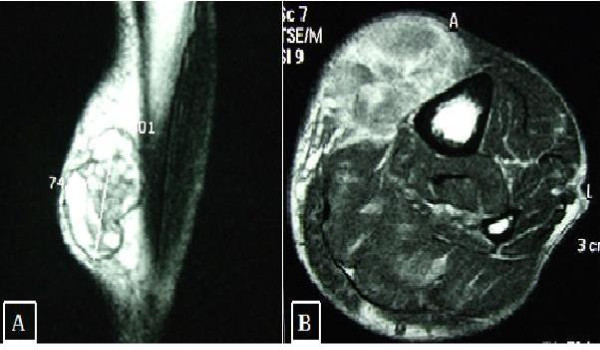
MRI images of the lesion. A) Sagittal TSE sequence with deletion of the T2 signal from adipose tissue. B) Axial MRI performed with T2 gradient-eco sequence.
